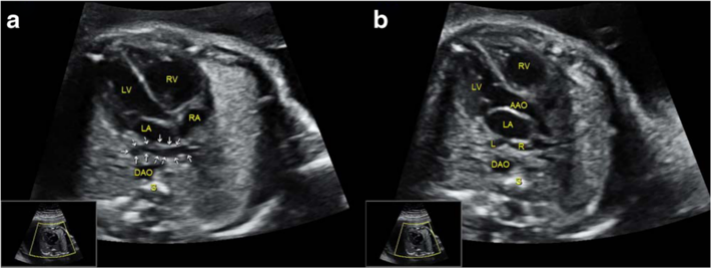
Sonographic images of a 22-gestational-week fetus diagnosed with TAPVC. In the 4CV, a tubular-like structure was detected between the LA and DAO (a). Gradual rotation of the ultrasound beam; two tiny structures were visualized to drain into the tubular-like structure (b). This was suspected to be TAPVC by screening sonographers under Protocol B, for whom this characteristic sign had been made evident. 4CV, four chamber view; AAO, ascending aorta; DAO, descending aorta; L, left; R, right; LA, left atrium; LV, left ventricle; RA, right atrium; RV, right ventricle; S, spine. The areas indicated by the *white arrows* depict the tubular-like structure
